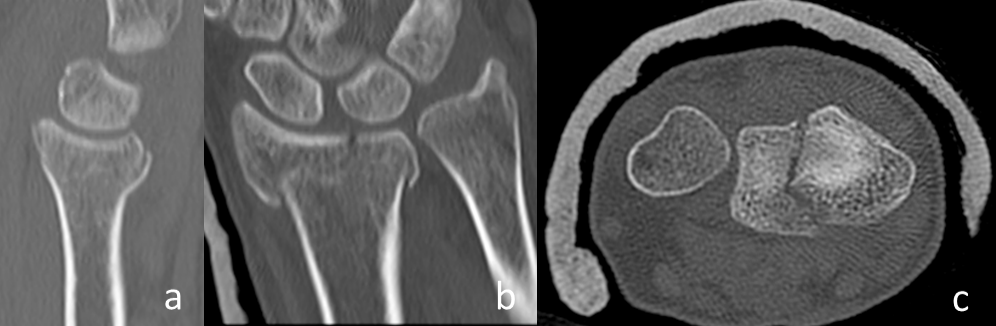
a: CT in sagittal plane showing the palmar cortex is not displaced. b: CT in frontal pane showing a sagittal split of the articular surface. c: CT in axial plane showing a sagittal split of the articular surface. Distal radius fracture (AO type C2) with a non-displaced palmar cortex (i.e. no palmar cortex) and no frontal split.
